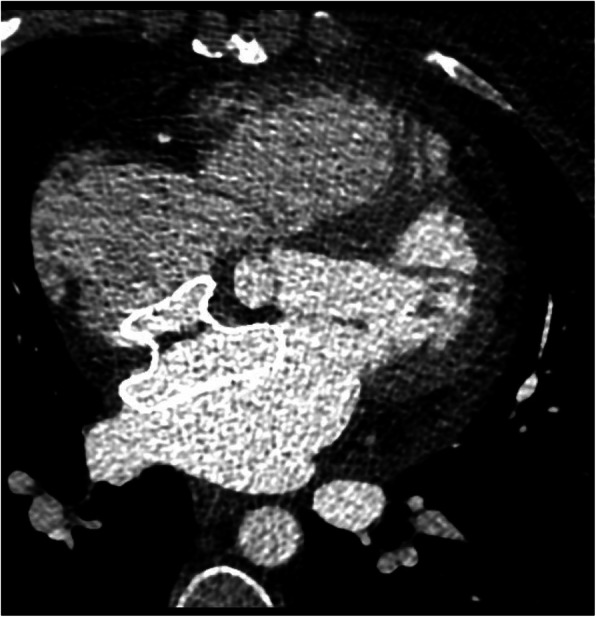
CTA showed no evidence of cardiac erosion or perforation
